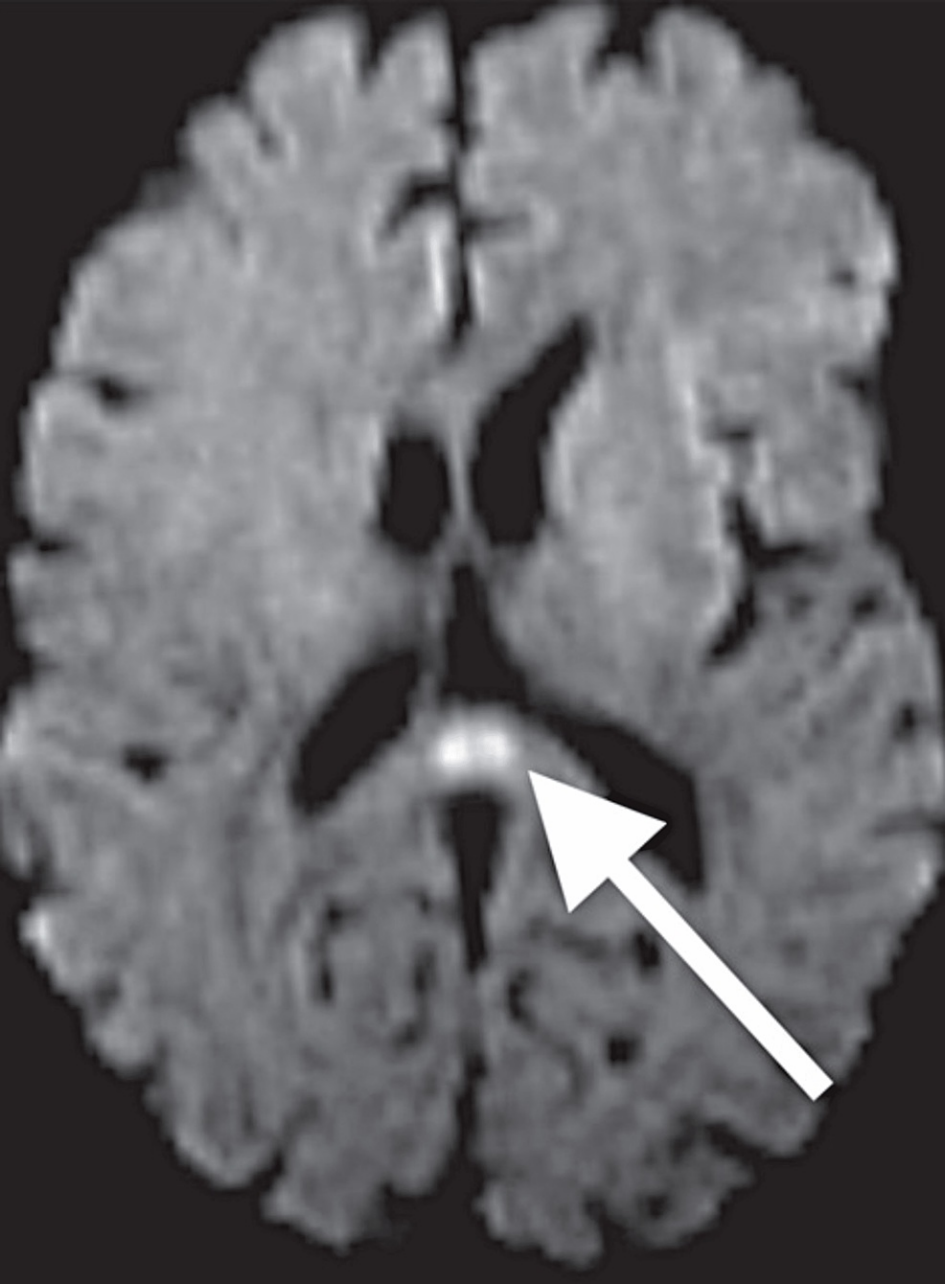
MRI of the brain showing diffusion weighted imaging restricted diffusion of the splenium of the corpus callosum
